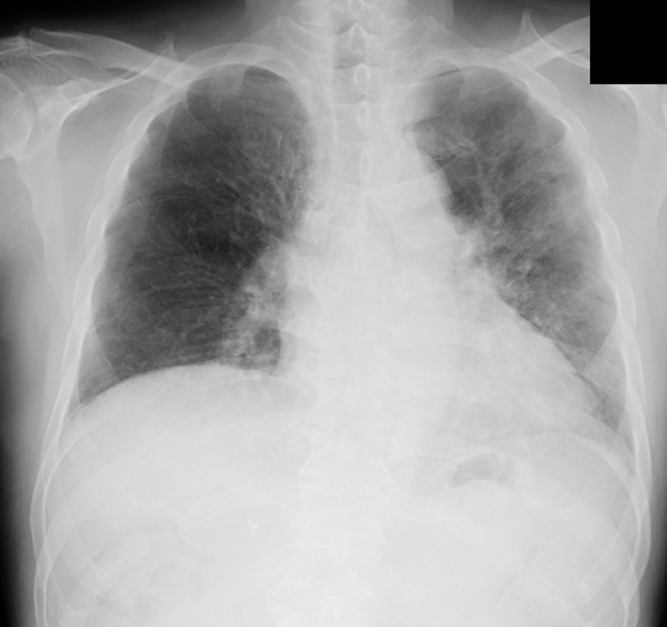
Chest X-ray on admission. Chest radiography revealing a cardiothoracic ratio of 55%, sharp right and dull left costophrenic angles, and diffuse ground-glass opacities in the right lung field.
